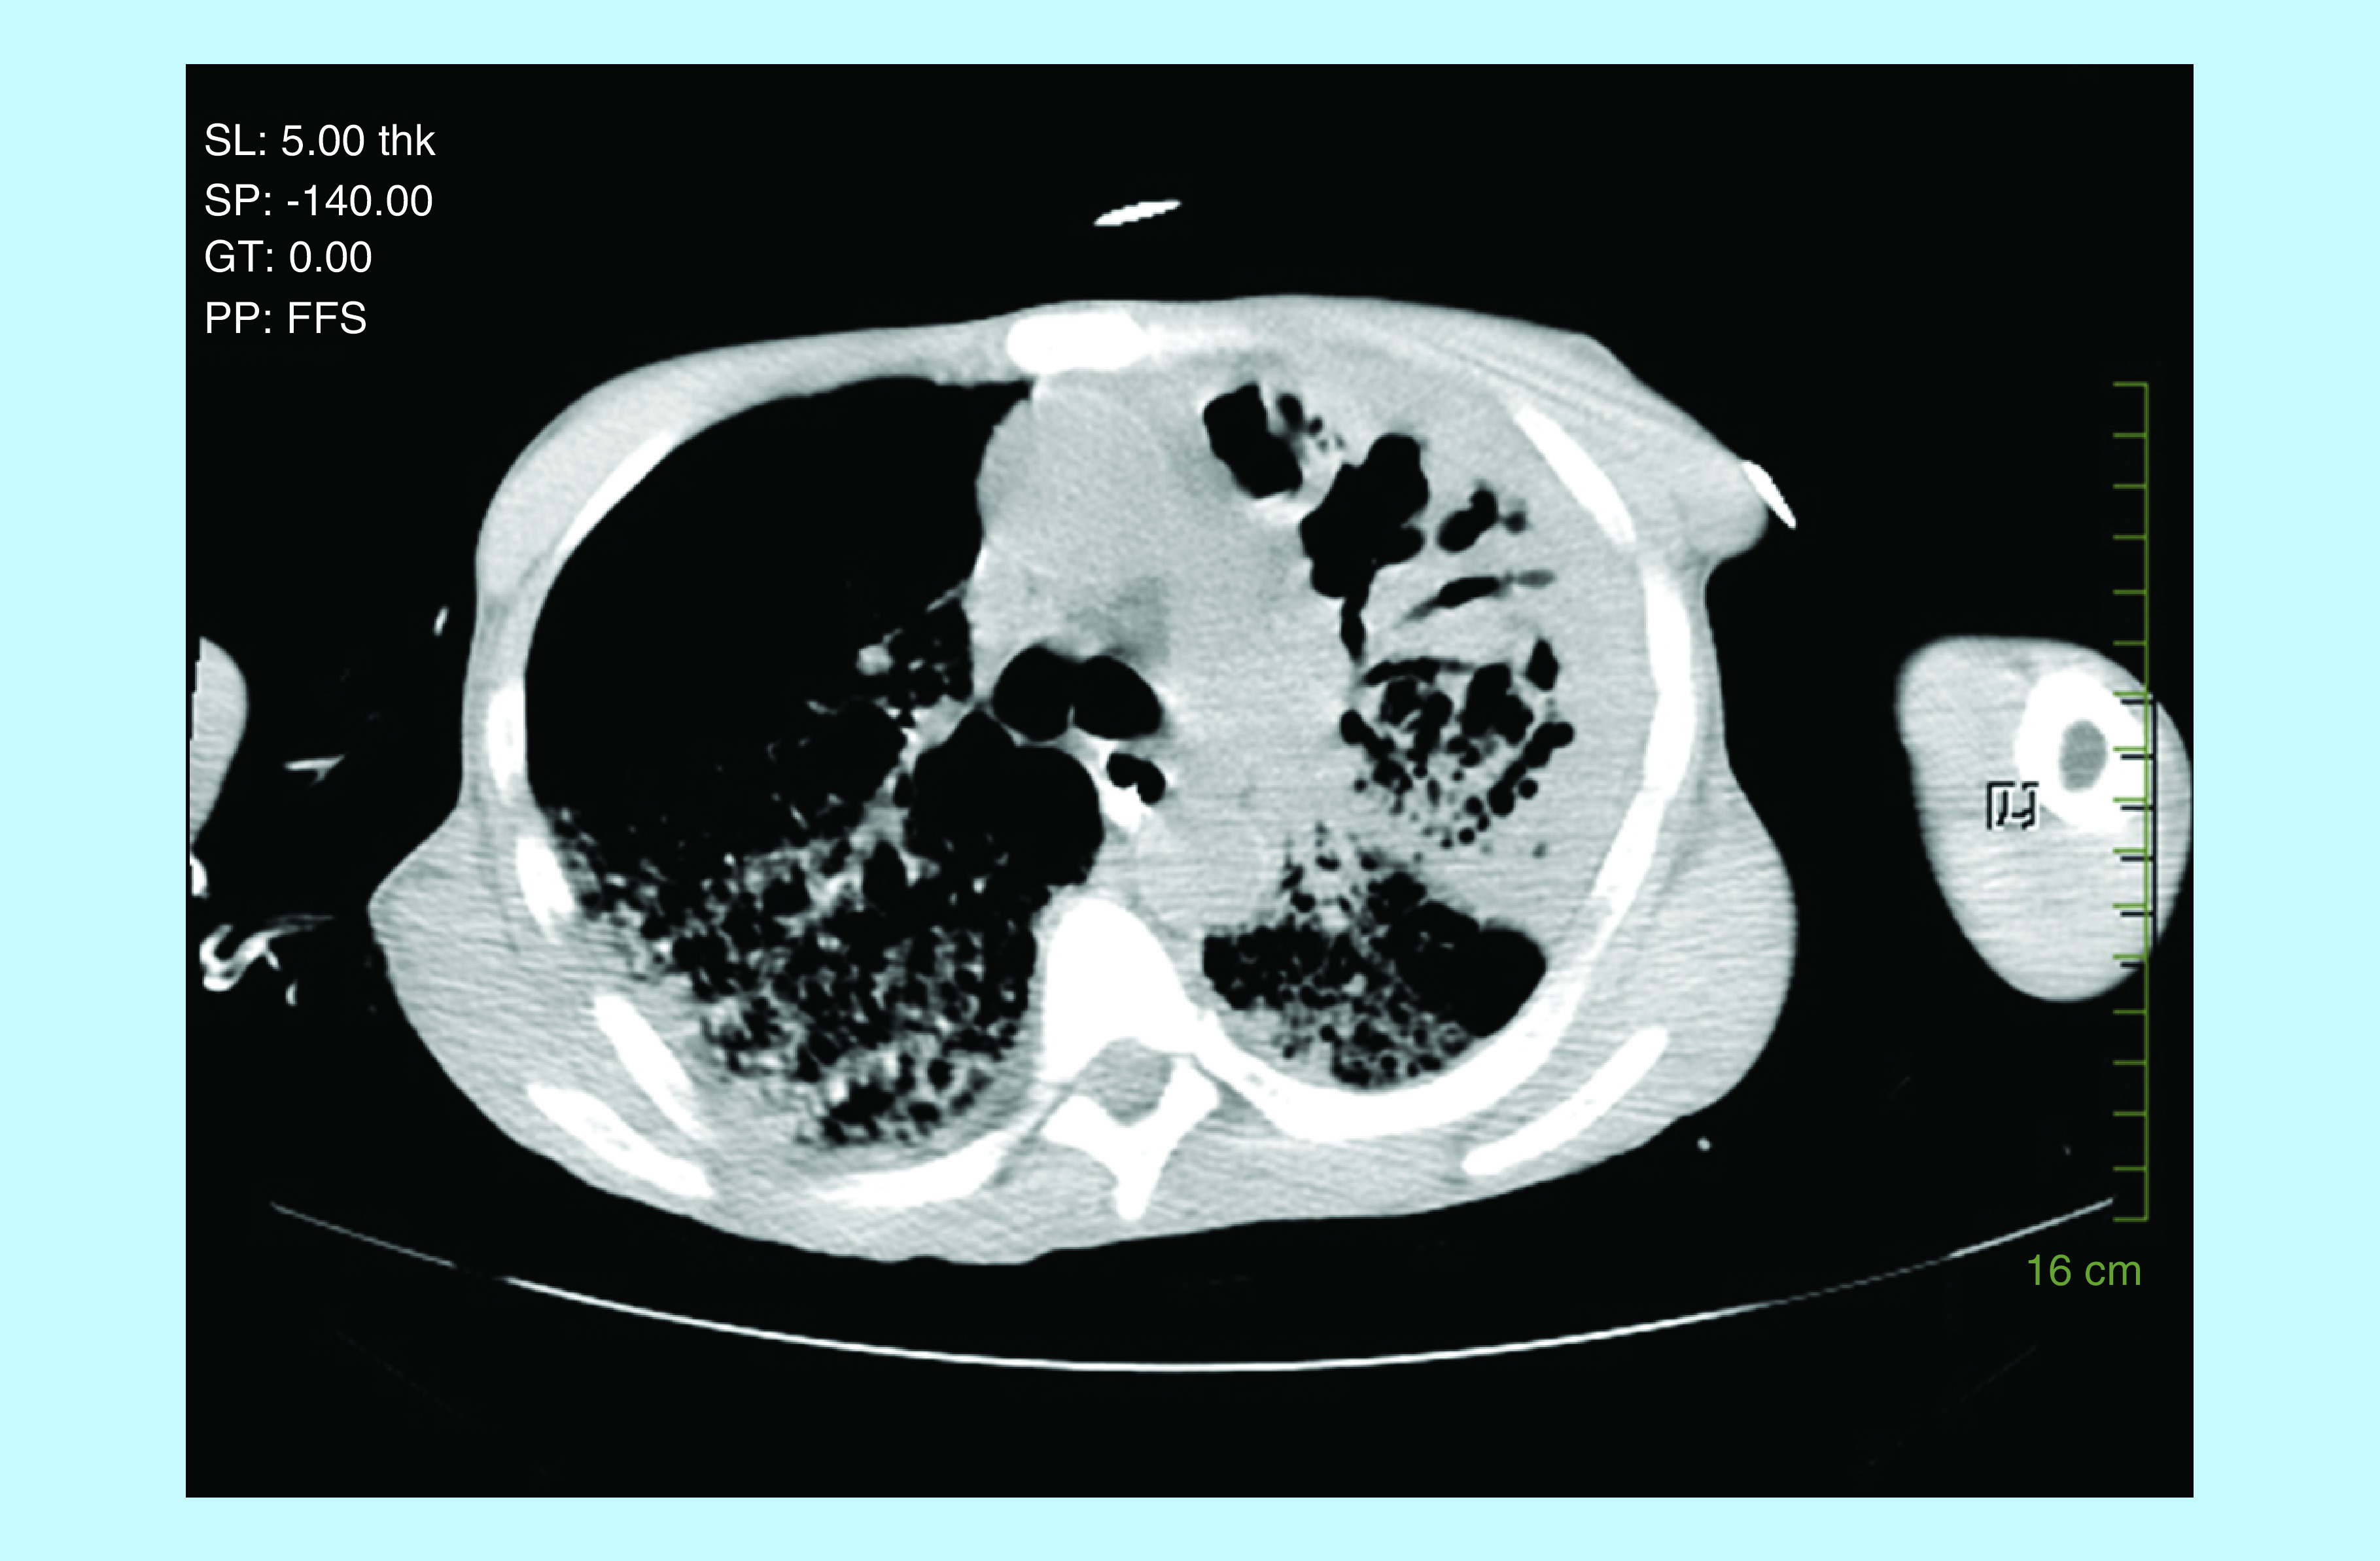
CT scan of the patient with tuberculosis and acute respiratory distress syndrome.
